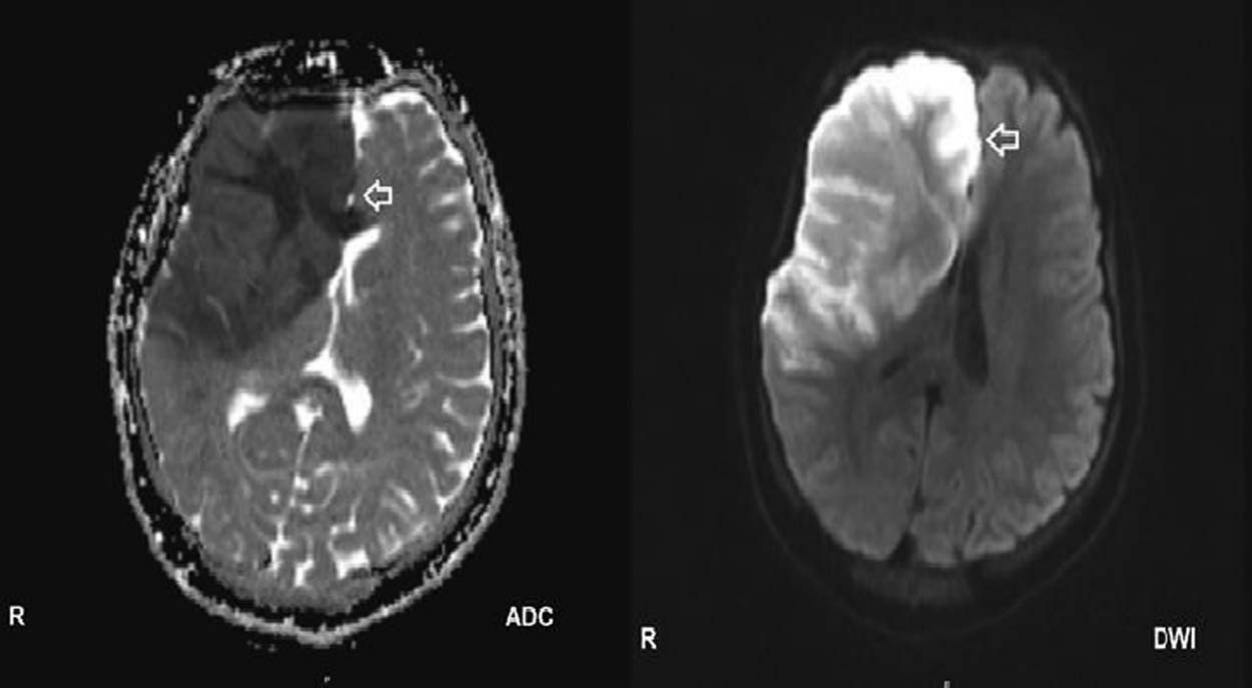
An axial head MRI utilizing diffuse weighted imaging (DWI) reveals a significant area of hyperintensity in the fronto-parieto-temporal lobes, along with hypo-intensity observed on the ADC map. This pattern is highly indicative of neuronal death and is commonly associated with acute infarction in the right MCA (middle cerebral artery) and ACA (anterior cerebral artery) regions.
